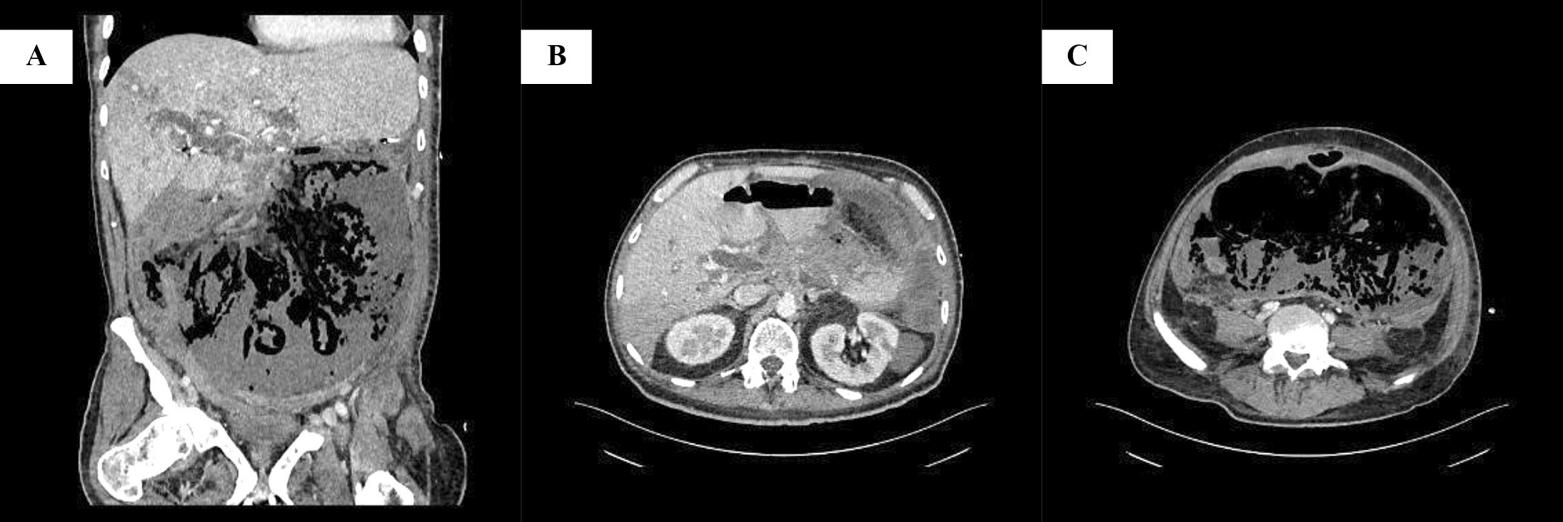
Preoperative computed tomography findings. A: Coronal view. Large fluid and air collection inside the peritoneal cavity, surrounded by thickened peritoneum with mild enhancement, due to peritonitis following bowel perforation; diffuse bowel wall thinning with intramural gas bubbles, suggestive of mesenteric ischemia; periportal edema and small peripheral area of hypoattenuation in the liver. B: Transverse view at the level of superior mesenteric artery origin. Extensive hypoattenuating mass located predominantly on the pancreatic body and less on the head, with invasion of the superior mesenteric artery from the level of its origin from the aorta; complete absence of attenuation of the superior mesenteric artery due to occlusion; the lesion extends to the hepatoduodenal ligament and porta hepatis; and dilatation of common bile duct. C: Transverse view at the level of L5. Large collection of fluid and gas in the peritoneal cavity, with thickened peritoneum; and thinning and absence of definition of the bowel wall, due to acute mesenteric ischemia.
